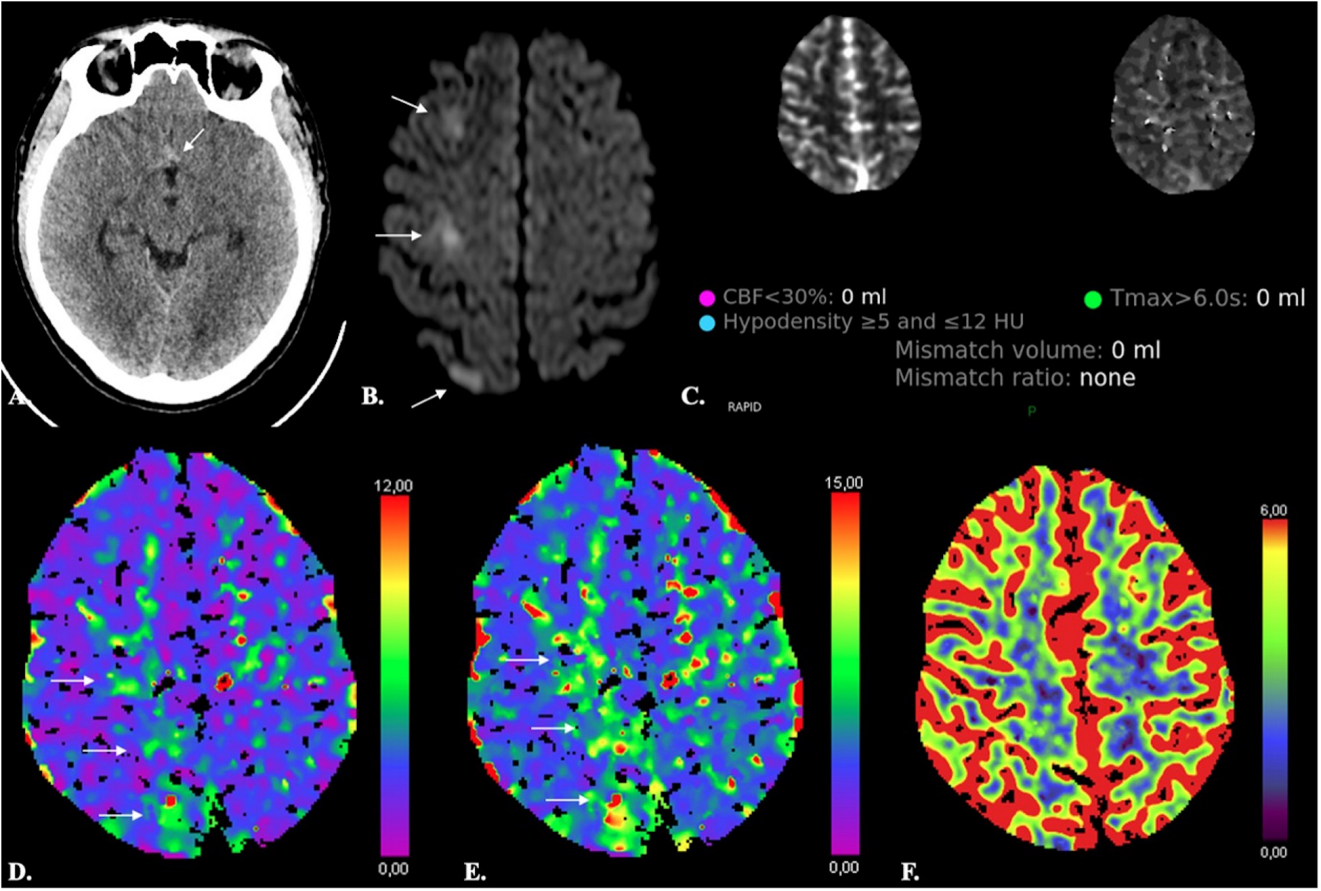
Limited sensitivity of CTP in detecting small ischemic lesions. A 38-year-old male with Fisher 1 aSAH (*arrow in A*) due to rupture of an ACom aneurysm treated with endovascular coiling developed abrupt onset paresis of the left inferior limb on day 8 after aSAH. Automated results of both the RAPID (C) and SyngoVia (*not shown*) CTP software did not reveal any significant decrease of CBF or CBV (F) and no significant increase of Tmax. On inspection, however, Tmax and TTD were moderately increased (*arrows in D and E*). Diffusion-weighted MRI (A) revealed three foci of acute ischemia in the right ACA territory (*arrows in B*).
